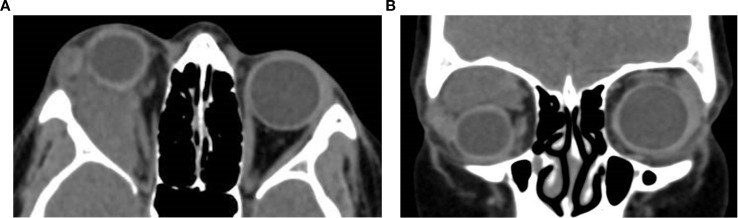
Axial (a) and coronal (b) contrast-enhanced CT images of an 11-year-old female patient demonstrate a right orbital soft tissue mass that is heterogeneous and poorly circumscribed. The lesion involves the extraconal space and extends medially with moderate displacement of the globe. The mass appears hyperdense relative to orbital fat and is associated with mild proptosis. The lacrimal gland appears separate from the mass with a preserved fat plane. There is no definitive evidence of calcification or bone erosion. It was surgically proven as rhabdomyosarcoma.
